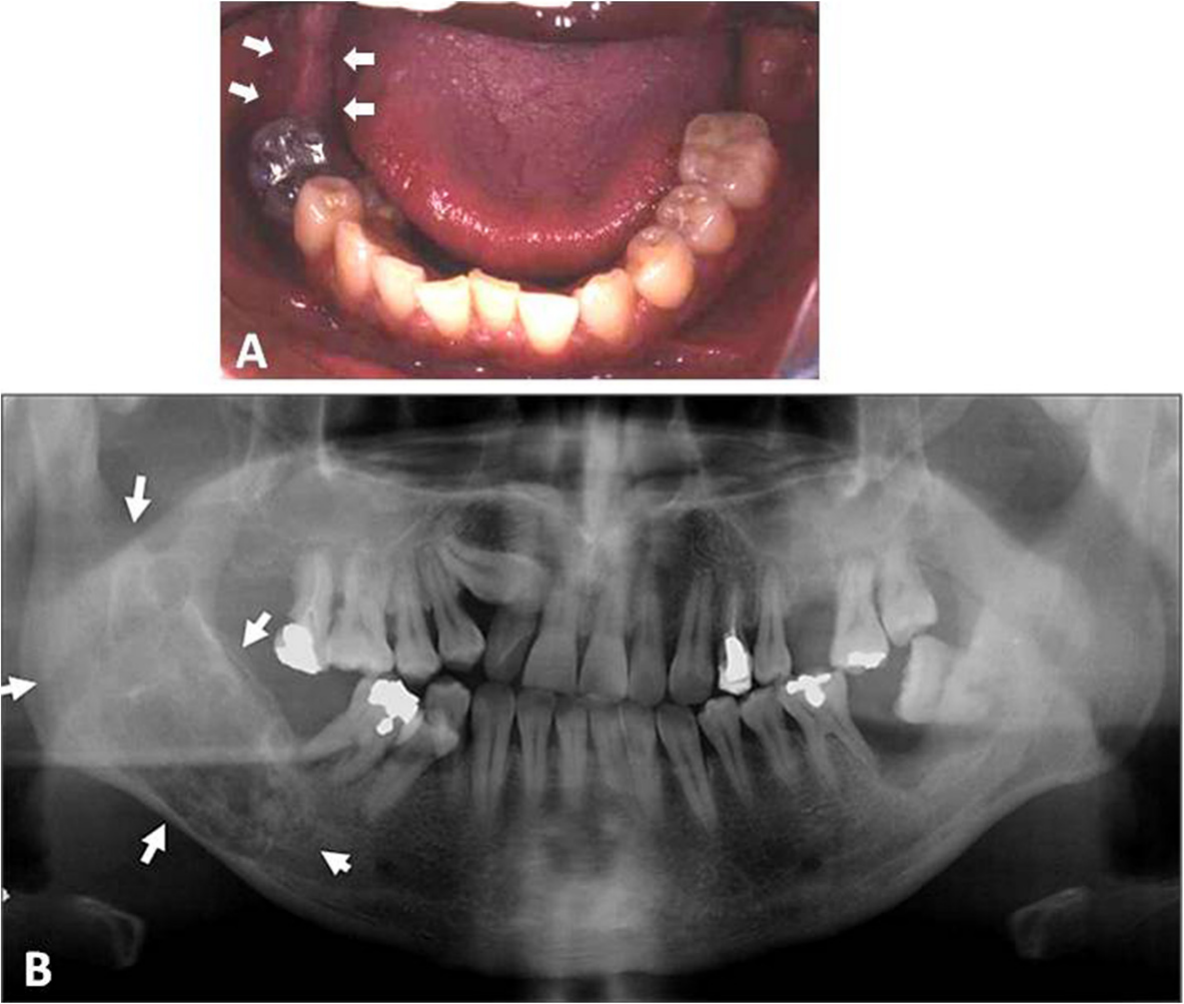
Pre-operative findings: (A) Intraoral photograph showing no swelling (arrows). (B) Panoramic radiograph indicating a “soap-bubble” appearance of the right jaw (arrows).
